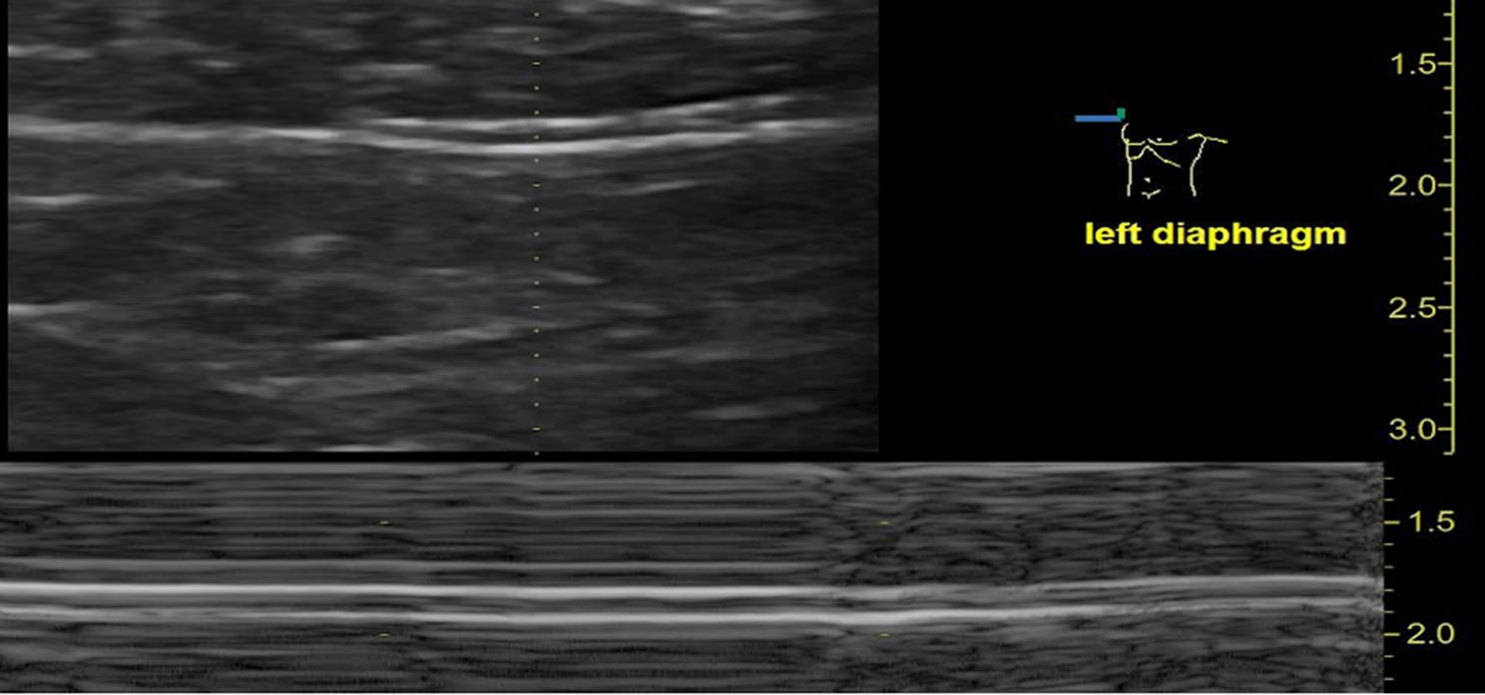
Ultrasound of the left diaphragm: No diaphragmatic thickening and elevation was detected
